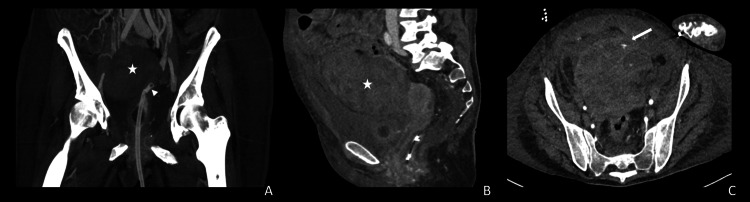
Abdominal CT scan. A) Maximal intensity projection on a coronal plan. B) Portal phase - sagittal plan . C) Arterial phase - axial plan. These demonstrate the bladder perforation due to the foley catheter (white arrowhead) leading to active arterial bleeding (white arrow) and a significant extra-peritoneal hematoma (white star).
